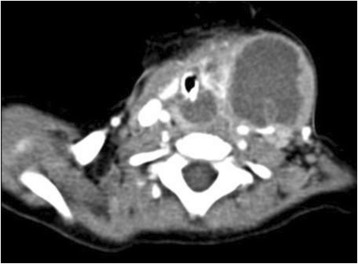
Axial enhanced CT showing involvement of the thyroid gland with surrounding multiloculated abscess
